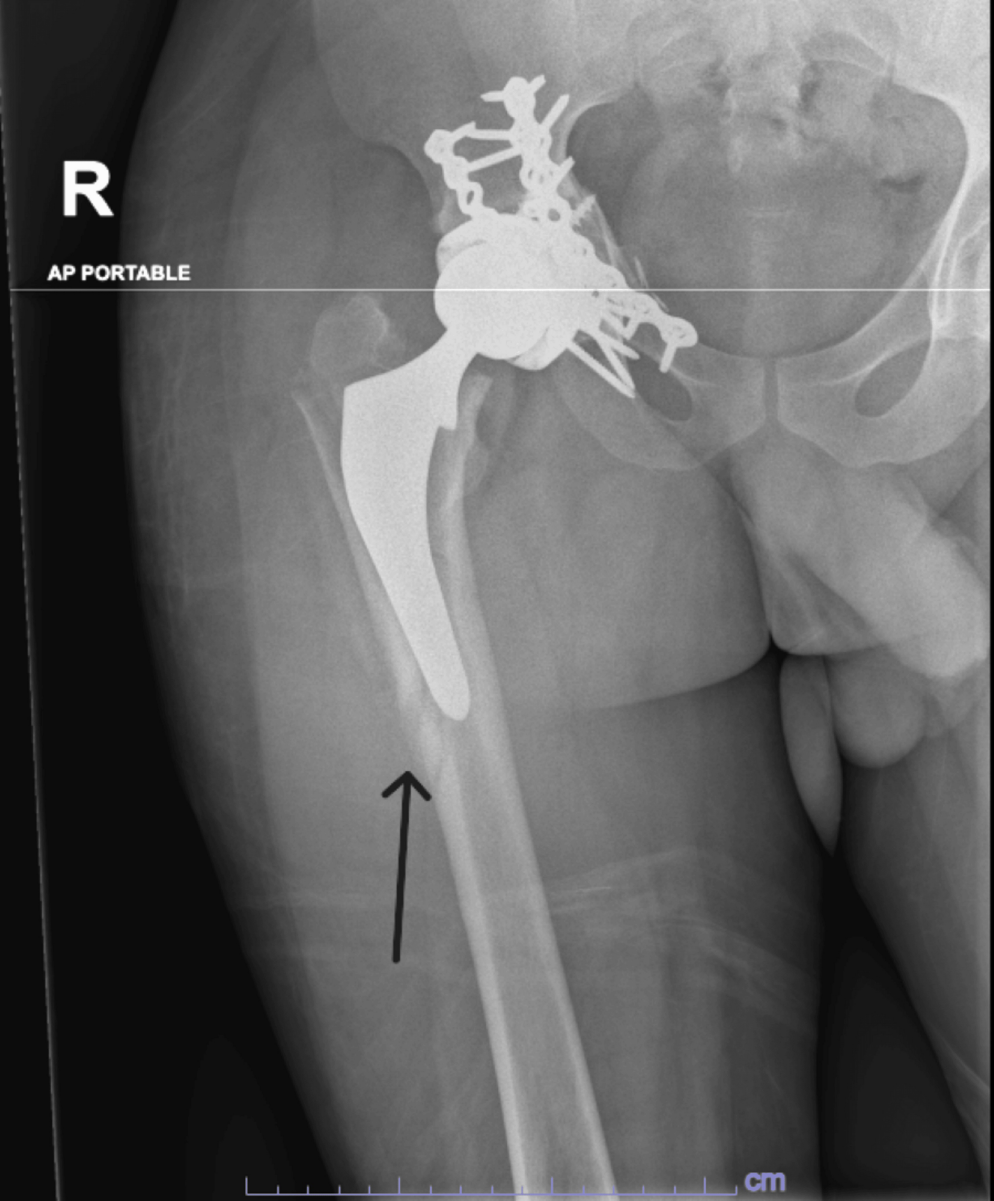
Preoperative AP X-ray of the right hip demonstrating periprosthetic fracture of the femoral shaft Arrow indicated fracture around the prosthesis. AP, anteroposterior
